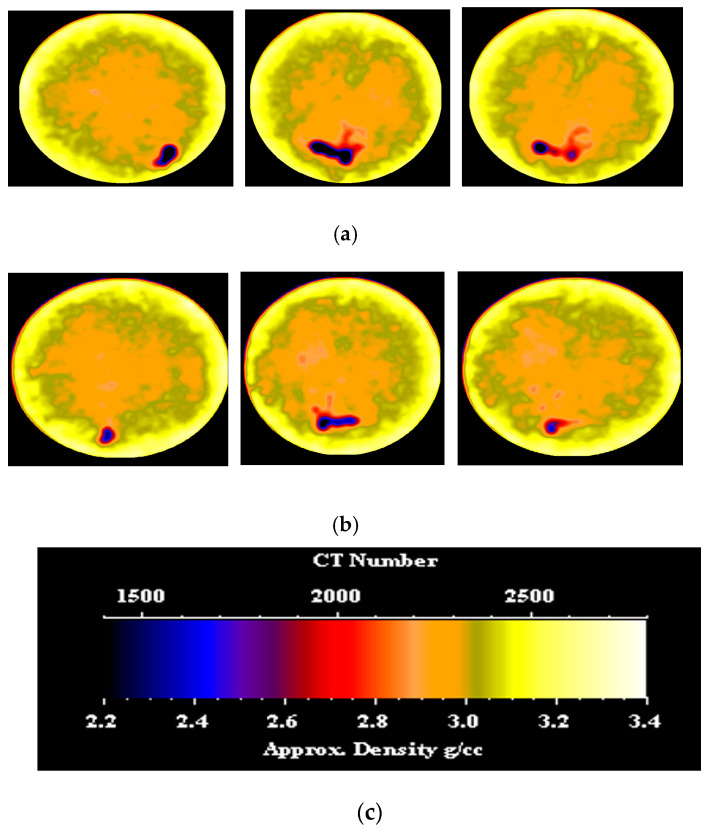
(a) CT scan of the induced channel before nano-silica gel treatment; (b) CT scan of the induced channel after nano-silica gel treatment; (c) color code scale.
